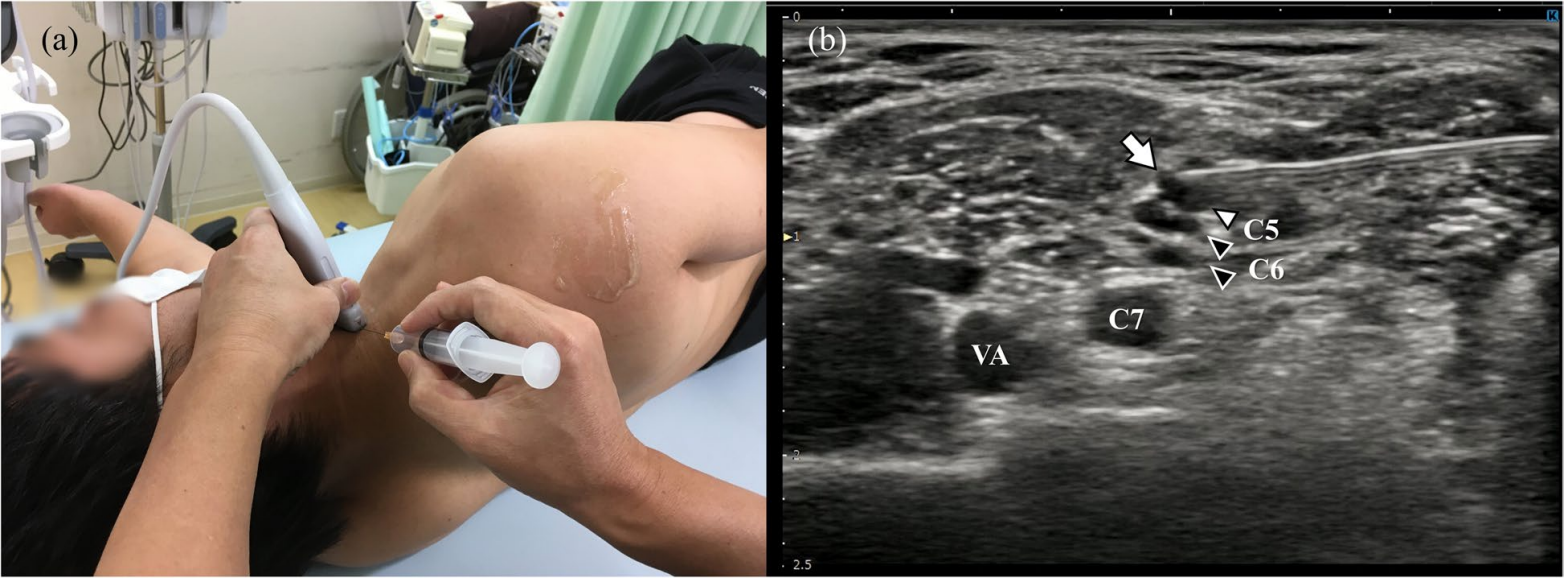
Ultrasound-guided C5 and C6 nerve root block. a With the patient in the half side-lying or lateral recumbent position, 12–15 mL of 1% mepivacaine is injected into the C5 and C6 nerve roots under ultrasound guidance. b Level where C5 and C6 form the truncus superior. The white arrowhead is C5, the black arrowheads are C6, and the white arrow is the needle tip. VA, vertebral artery
